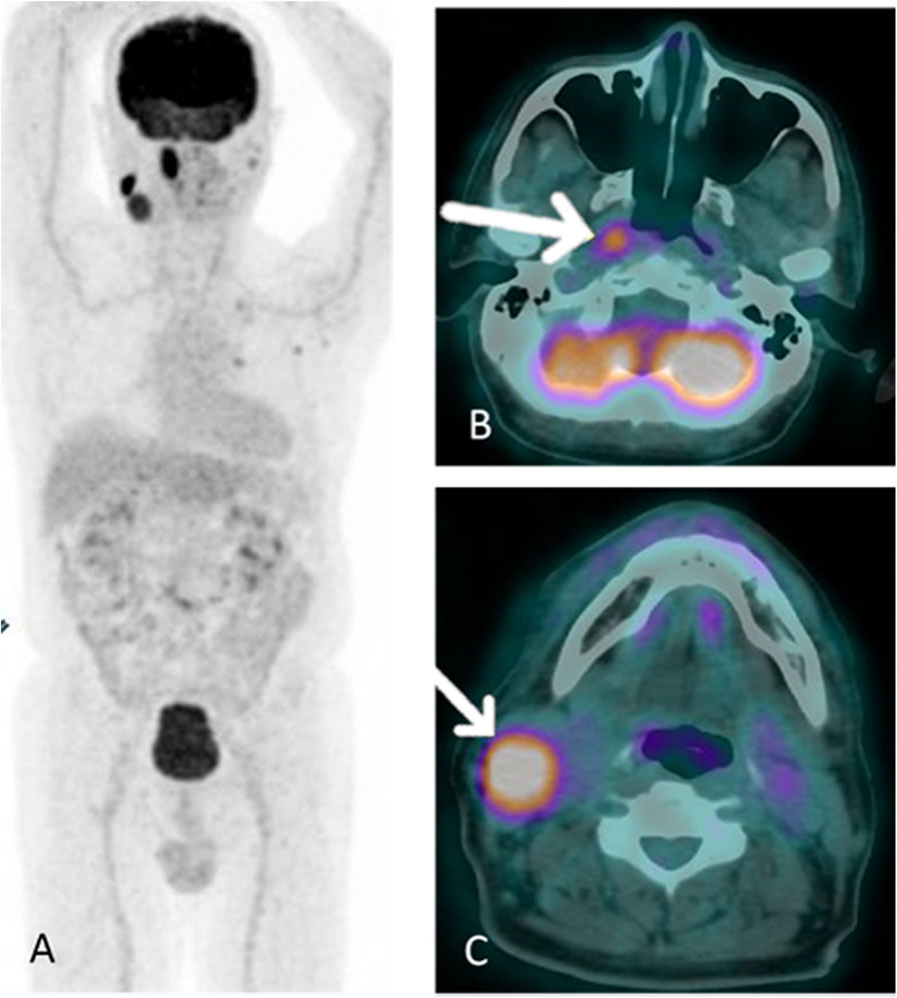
FDG PET/CT of patient number 1. Hypermetabolic lesions in several parts of head and neck. a MIP. b hypermetabolic lesion at right nasopharyngeal wall. c hypermetabolic enlarge lymph node at neck region
